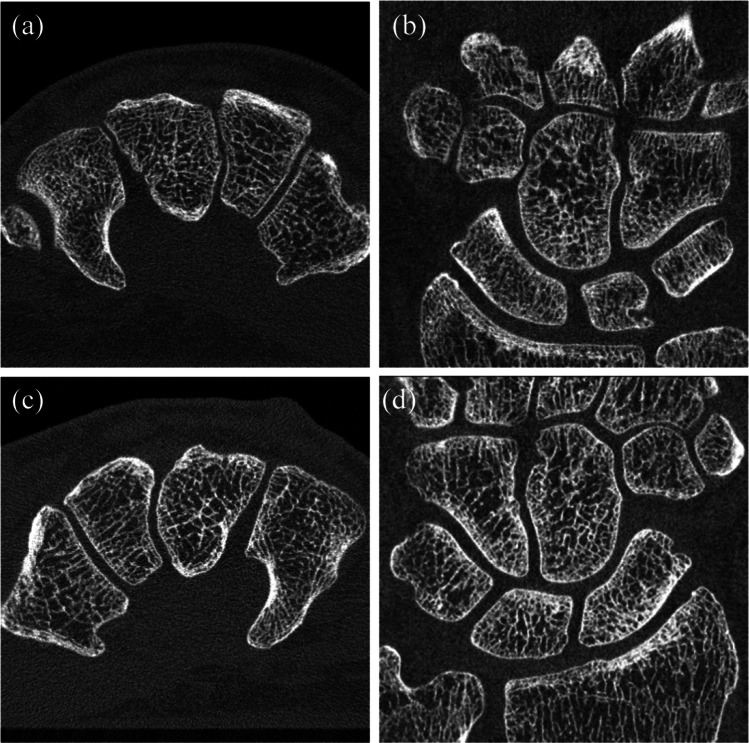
Multi-planar reformation (MPR) images of human extremity bones: (a) axial and (b) coronal, volunteer 1; (c) axial, volunteer 2; and (d) coronal, volunteer 2, with UHRCBCT. Scans were performed after the approval of the institutional ethics review board. The window width/center was set at 3000/700 HU
